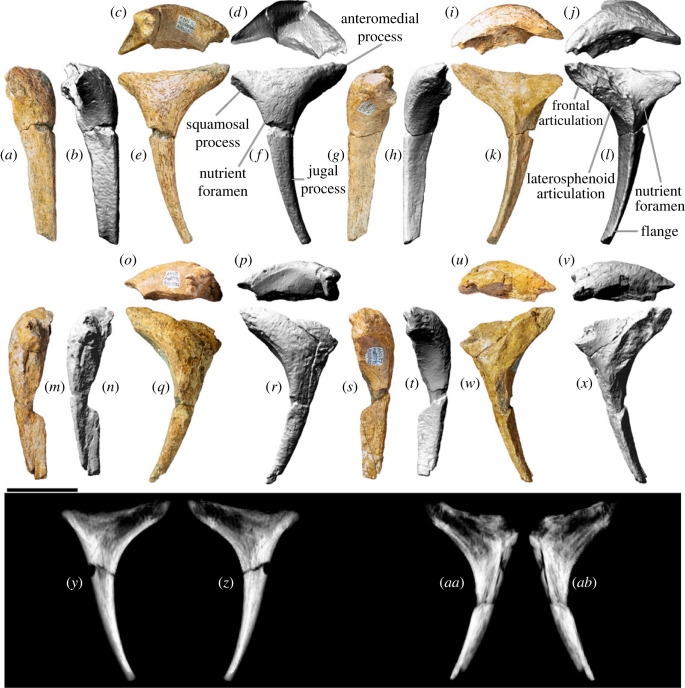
Diamantinasaurus matildae* referred postorbitals (AODF 0906). (*a–l*) Right postorbital in (*a,b*) posterior, (*c,d*) ventral, (*e,f*) lateral, (*g,h*) anterior, (*i,j*) dorsal and (*k,l*) medial views. (*a*), (*c*), (*e*), (*g*), (*i*) and (*k*) are photographs, (*b*), (*d*), (*f*), (*h*), (*j*) and (*l*) are three-dimensional models derived from surface scans. (*m–x*) Left postorbital in (*m,n*) posterior, (*o,p*) ventral, (*q,r*) lateral, (*s,t*) anterior, (*u,v*) dorsal and (*w,x*) medial views. (*m*), (*o*), (*q*), (*s*), (*u*) and (*w*) are photographs, (*n*), (*p*), (*r*), (*t*), (*v*) and (*x*) are three-dimensional models derived from surface scans. (*y,z*) Three-dimensional digital models (derived from medical CT data) of the right postorbital in (*y*) lateral and (*z*) medial views. (*aa*–*ab*) Three-dimensional digital models (derived from medical CT data) of the left postorbital in (*aa*) lateral and (*ab*) medial views. Scale bar = 50 mm.
